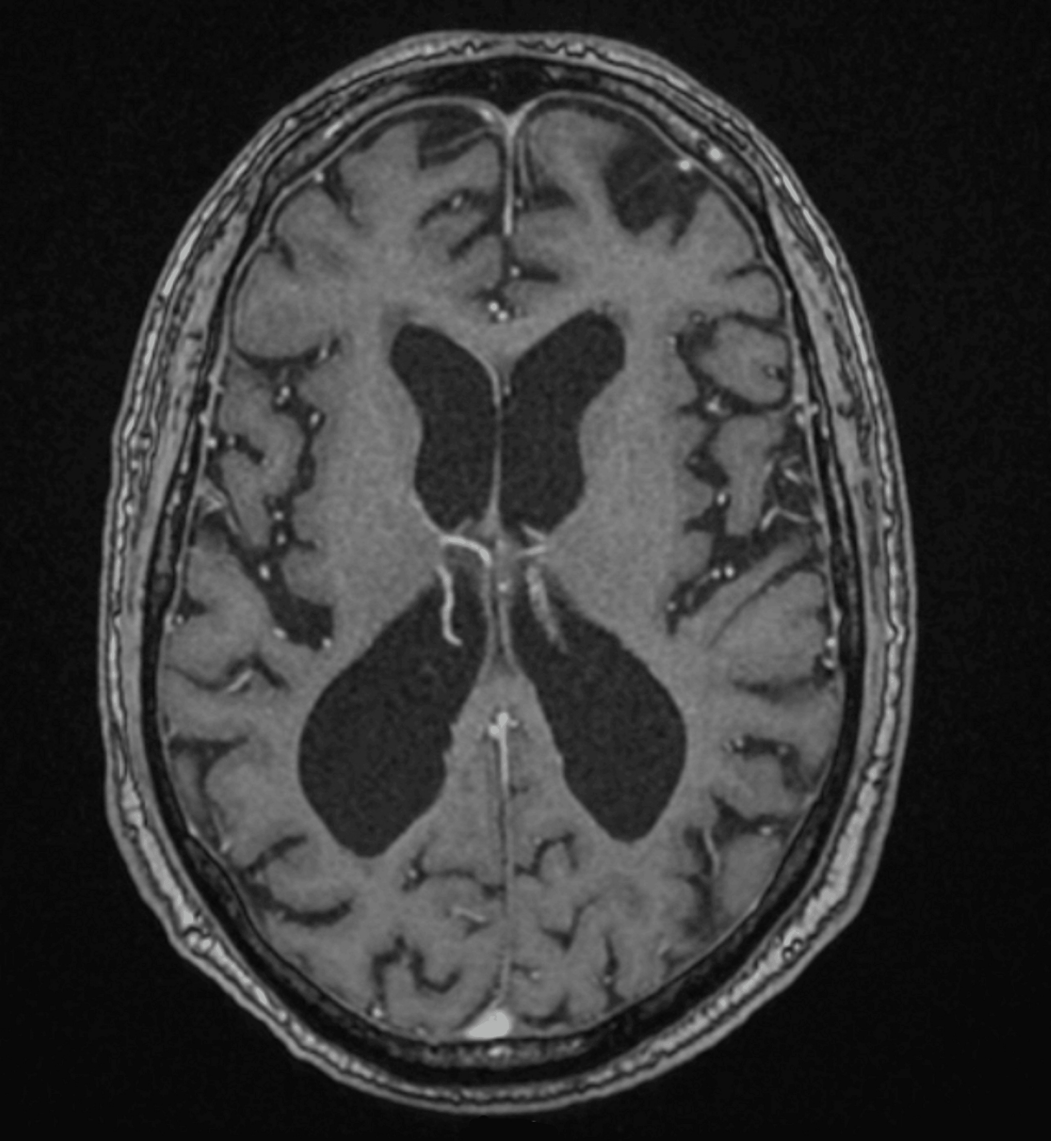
Axial T1-weighted brain MRI MRI: magnetic resonance imaging
